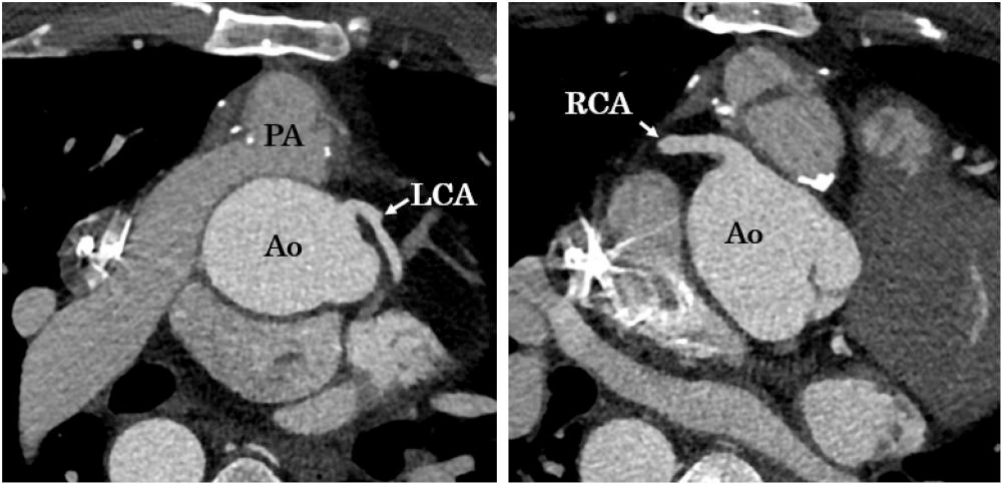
Contrast-enhanced CT scan. No coronary artery stenosis, occlusion, or pulmonary artery stenosis was shown. CT, computed tomography; LCA, left coronary artery; RCA, right coronary artery; Ao, aorta; PA, pulmonary artery.
